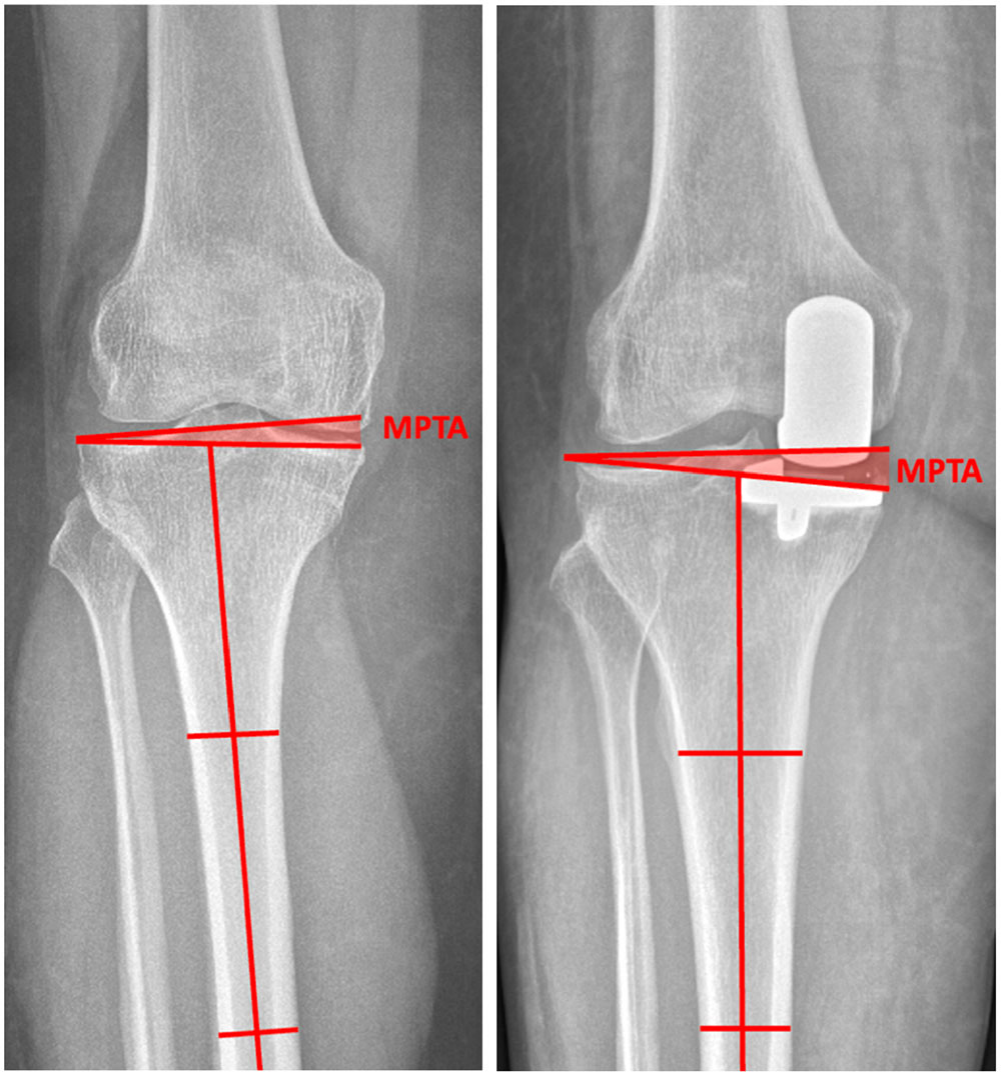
Measurements of MPTA. Left: MPTA anterior to posterior preoperative knee radiograph showcasing MPTA measurement. Right: MPTA anterior to posterior post‐operative knee radiograph showcasing MPTA measurements. MPTA, medial proximal tibial angle.
